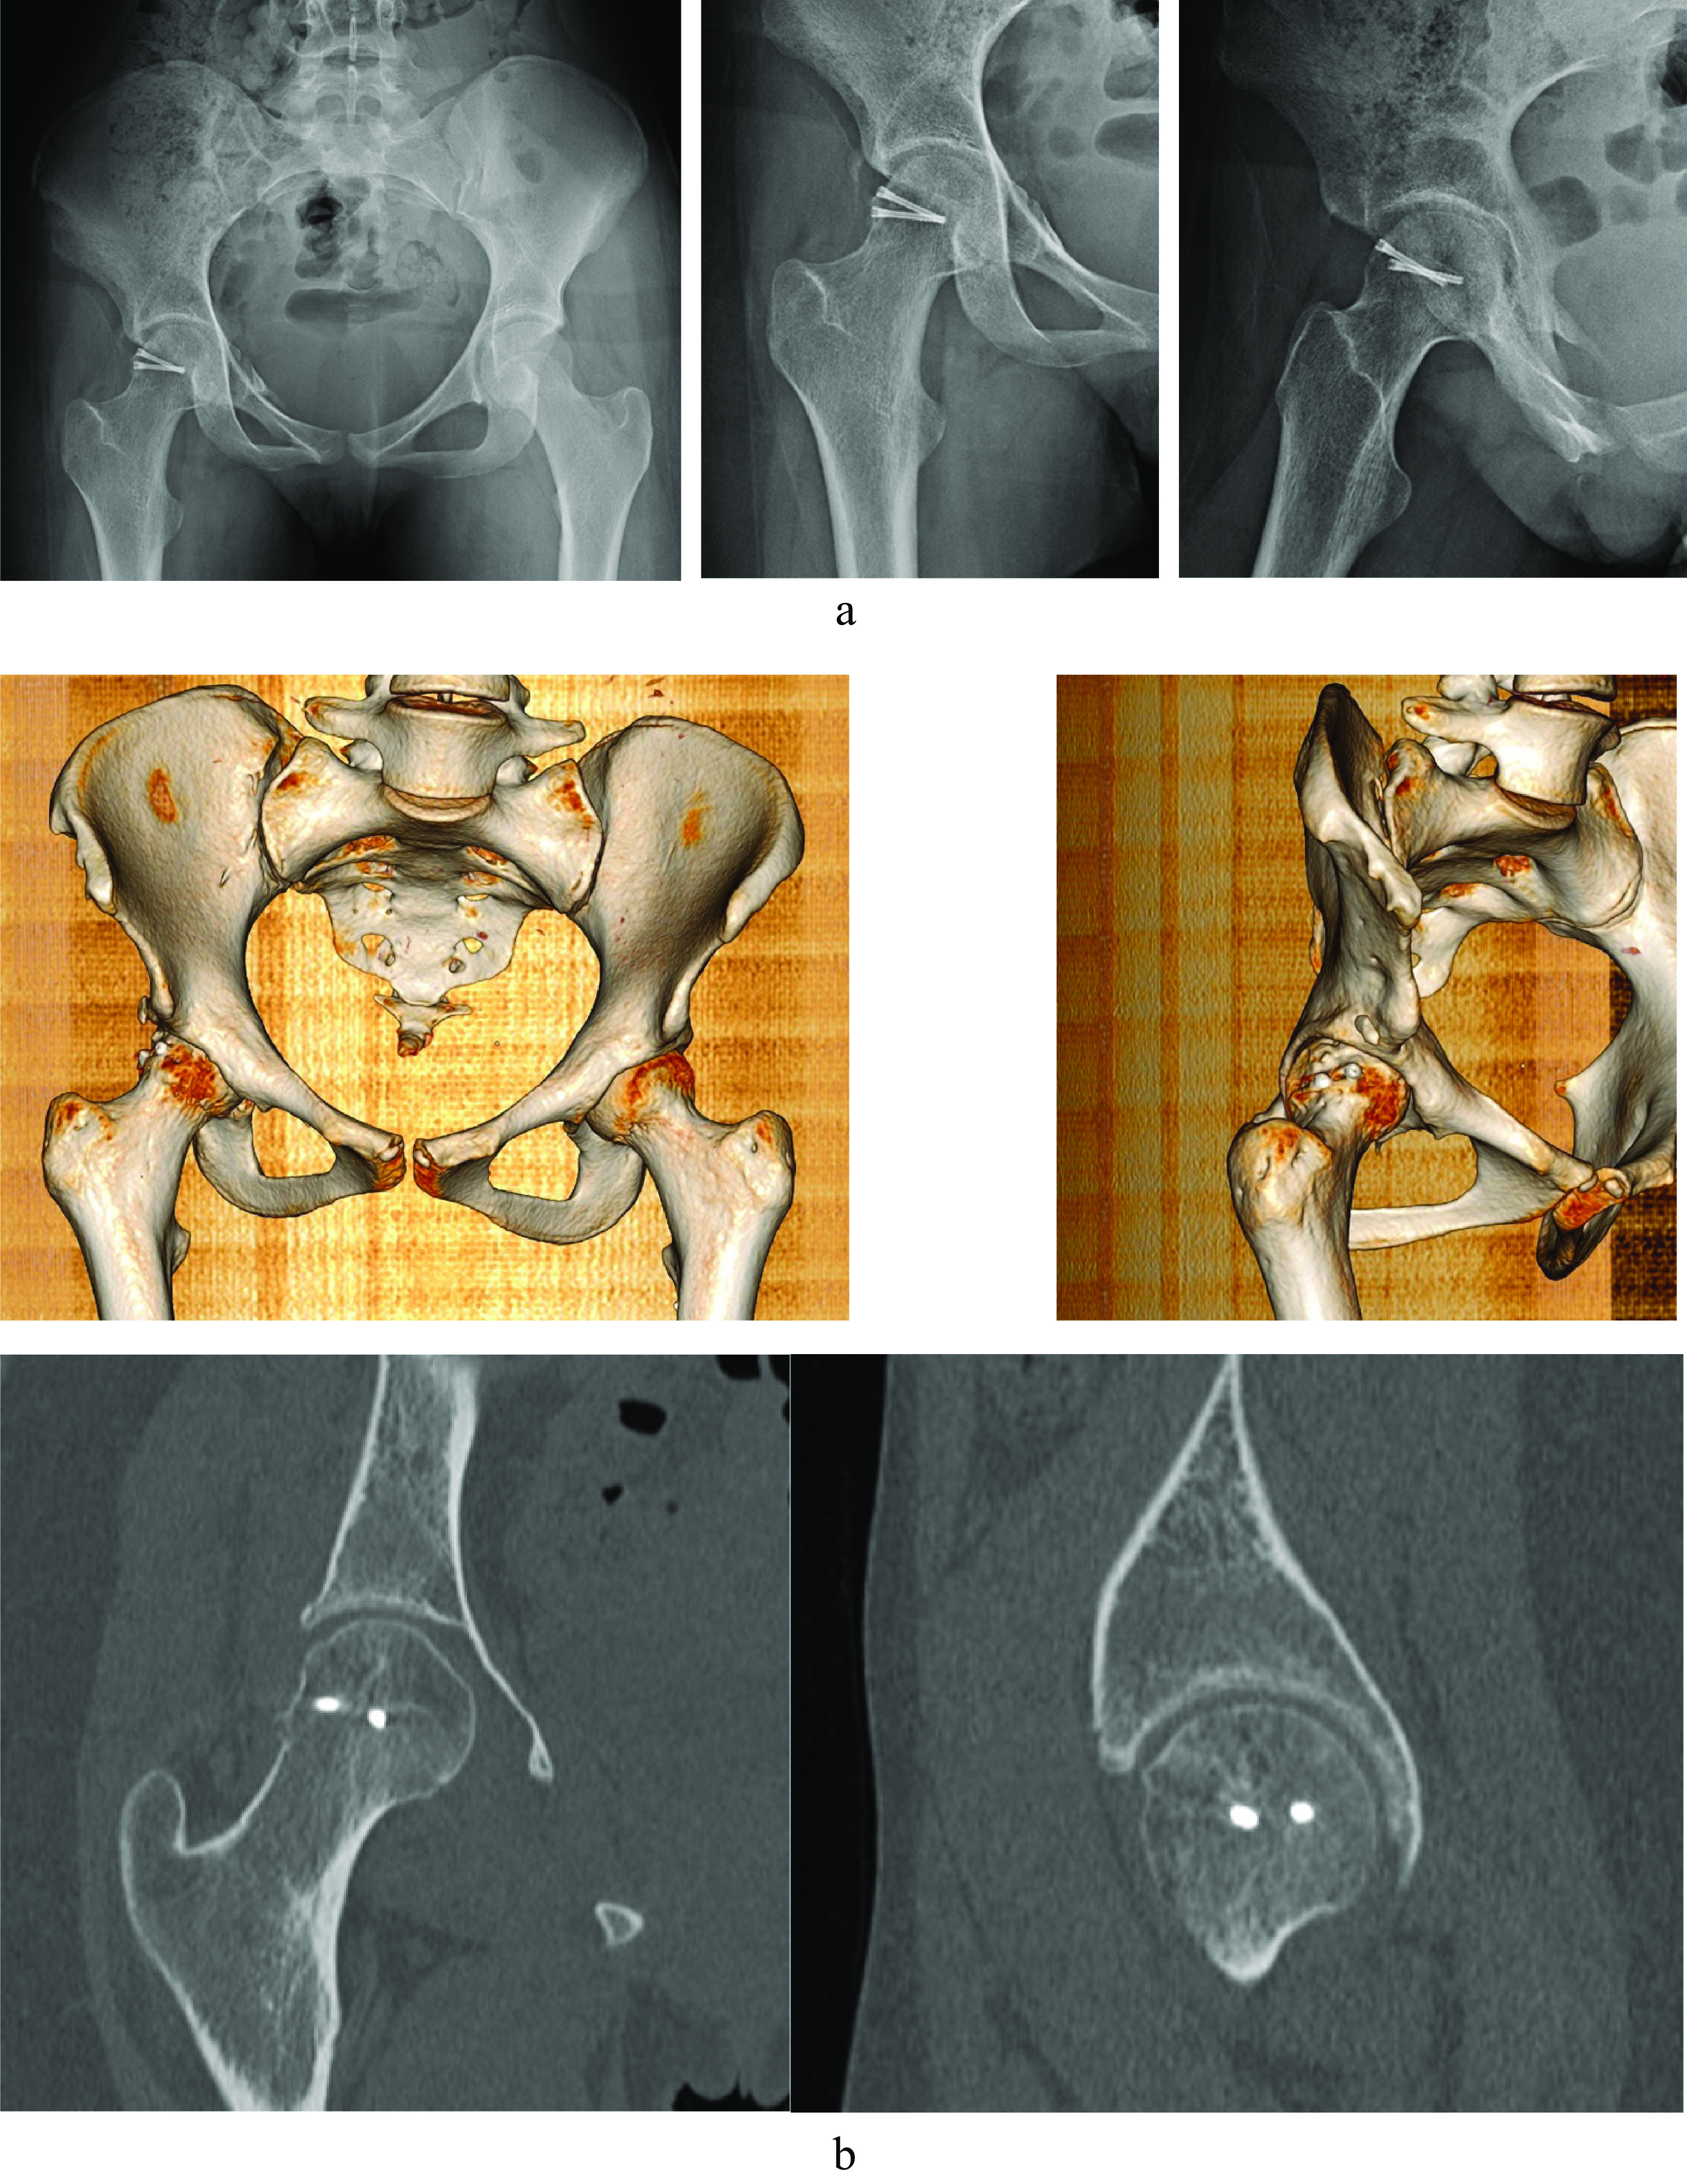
X-ray image of the right hip 3 months after surgery (a) *left*: pelvic AP, *middle*: right AP axial, *right*: external and internal oblique view (b) CT scan 1 year after surgery demonstrating both femoral head screws and a slightly irregular surface with good joint space at the upper part of the femoral head.
